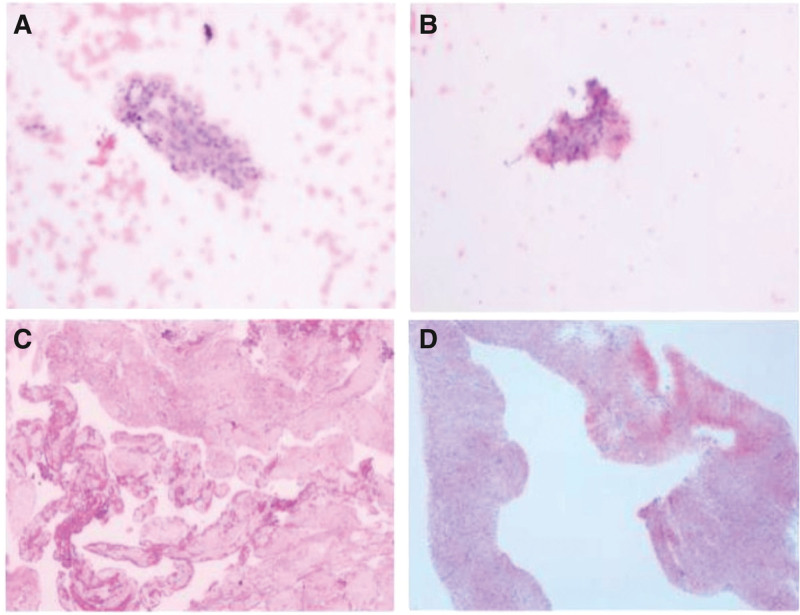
(A, B) Brush biopsy of the descending duodenum revealed the presence of a few round/short spindle cells. (C, D) CT-guided puncture biopsy of the abdominal mass was performed, and the pathological results indicated spindle cell hyperplasia.
